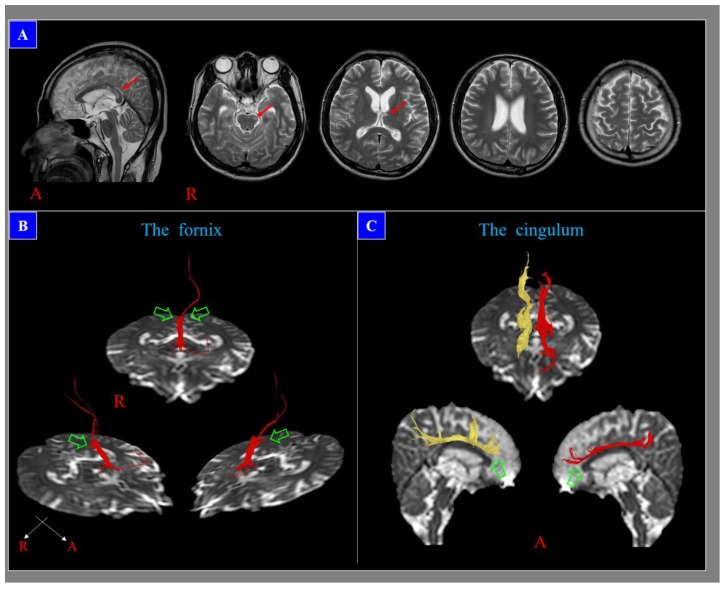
A 28-year-old male who showed loss of consciousness for ten days after a pedestrian–car accident, with diffuse axonal injury lesions (red arrows) in T2-weighted images (A). However, diffusion tensor tractography results reveal neural injuries (discontinuations (green arrows): both fornical crura (B) and anterior cingulums(C)) that are not related to these lesions; these neural injuries are consistent with the subject’s cognitive impairment, which developed after head trauma.
